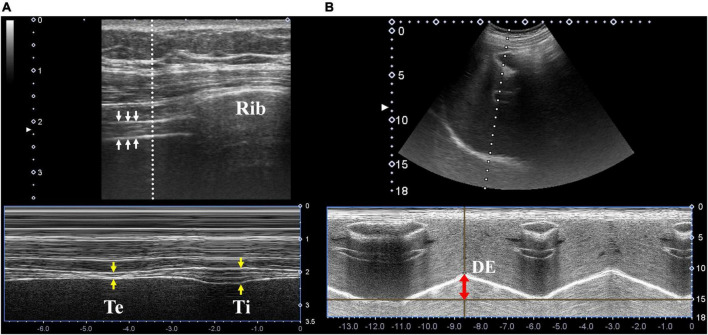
Diaphragmatic ultrasonography. (A) Ultrasonographic images of the diaphragm (white arrows) in the longitudinal plane were located in the right 7^th^ intercostal space along the mid-axillary line. The thickness at end expiration (yellow arrows, labeled as “Te”) and end inspiration (labeled as “Ti”) were measured using M-mode ultrasonography. (B) Ultrasonographic images of the diaphragm in the sagittal plane were located in the right subcostal area between the mid-clavicular and anterior axillary lines. Diaphragm excursion was measured using M-mode ultrasonography (red double-head arrow).
